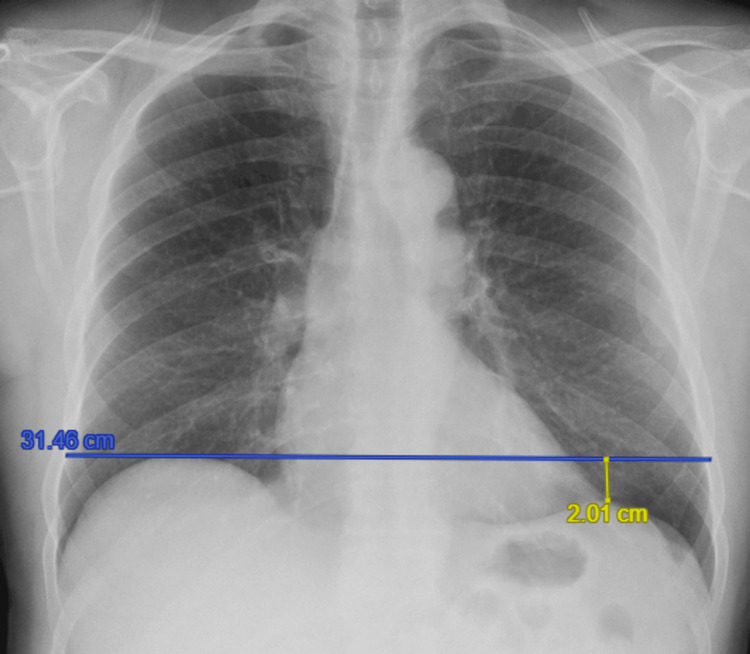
Chest X-ray in 2013, showing normal level of the right hemidiaphragm (blue line), when compared to the left hemidiaphragm (yellow line).
